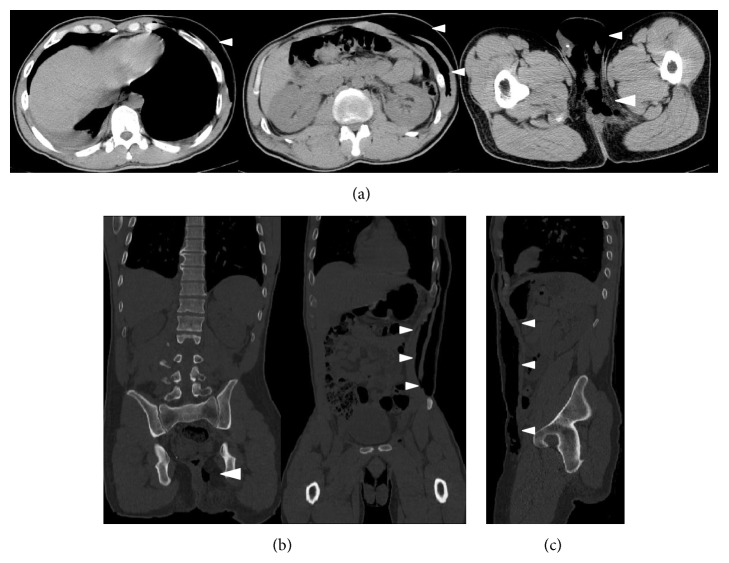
Abdominal CT on admission. Subcutaneous emphysema spreading from the scrotum to the left chest: plain CT (a), frontal section (b), sagittal section (c), and subcutaneous gas (arrow head).
